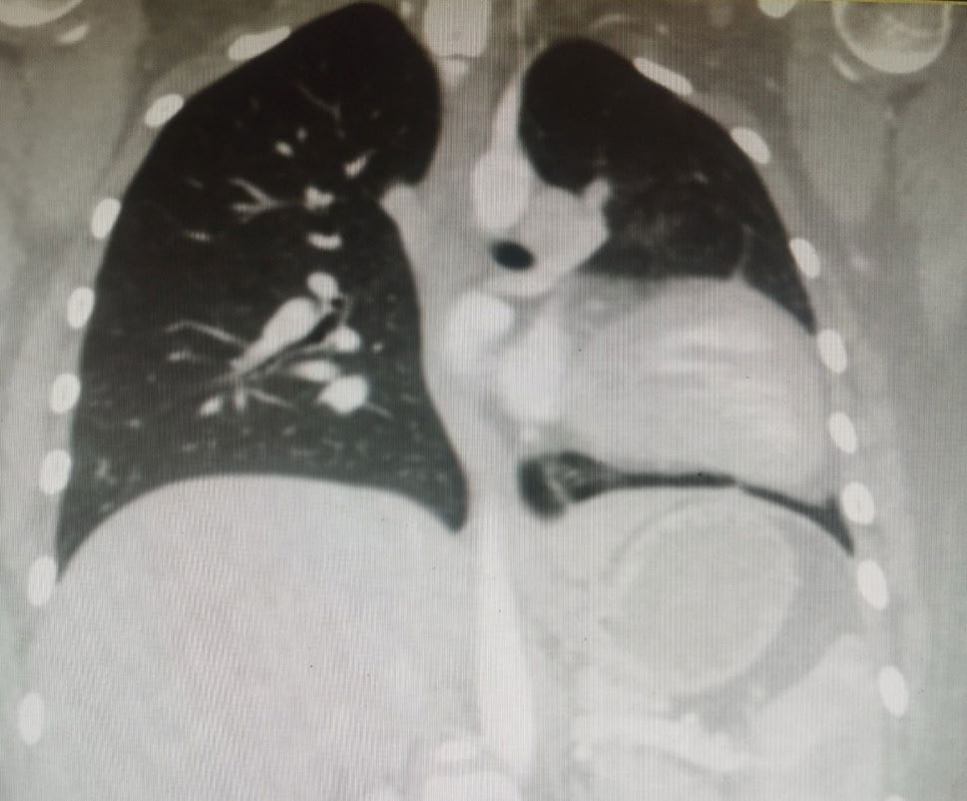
Chest CT Scan (Coronal View): Lung parenchyma is visible between the left hemidiaphragm and the base of the heart. Hyperinflation of the right lung and reduced volume of the left lung are noted
